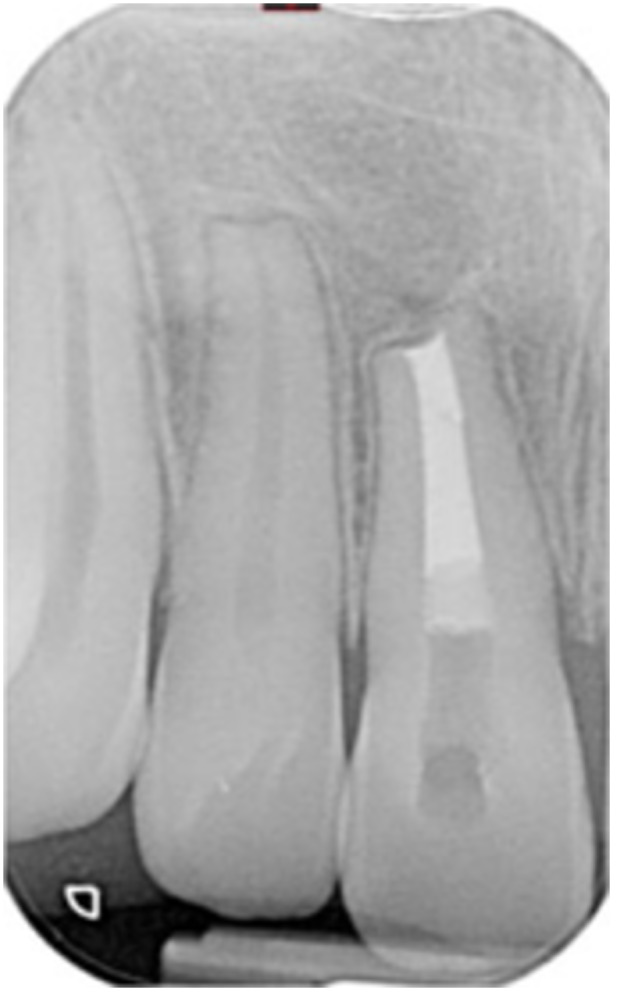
Case 11: The preoperative CBCT and periapical radiograph show an extensive periapical radiolucency associated with tooth 11, which has thinned the labial cortical plate and has caused displacement of tooth 12. Apical surgery was performed on tooth 11 following satisfactory nonsurgical root canal treatment. At the 6‐month follow‐up, complete healing is observed, and tooth 12 has returned to its upright position.
